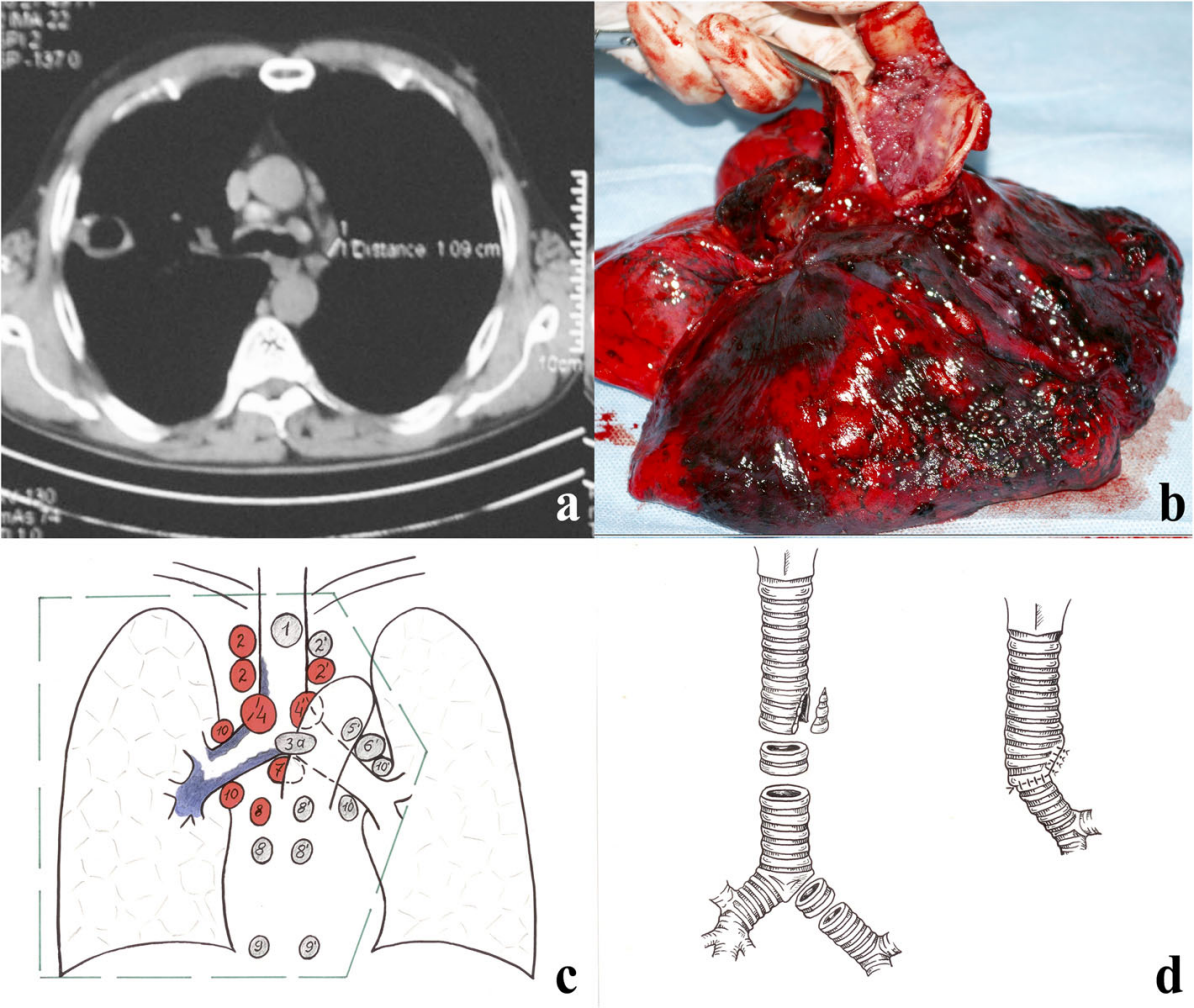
Clinical and imaging manifestation of the case. a CT scan prior to surgery (4.0 cm cavity in the right upper lobe with focal seeding; mediastinal lymph nodes enlargement); b Lung gross section; c Lymphadenectomy volume and metastasis spread; d Tracheobronchial reconstruction scheme
